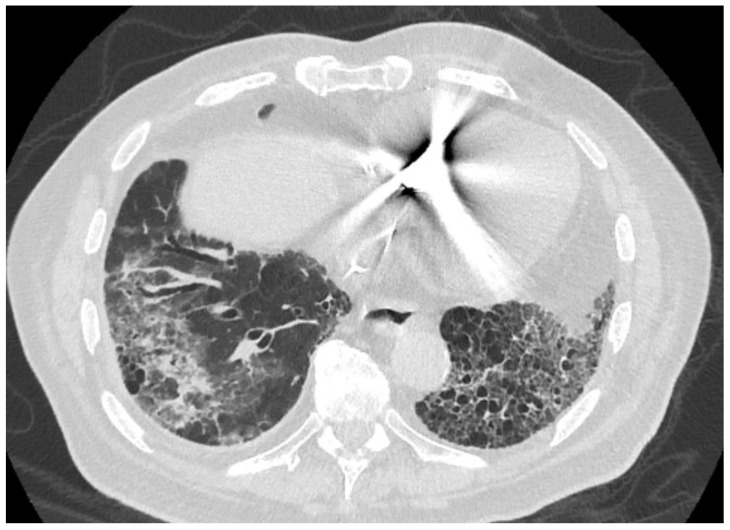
Axial view of chest computed tomography (CT) demonstrates typical honeycombing in the left lower lobe and traction bronchiectasis in the right lower lobe. The groundglass and consolidative opacity seen in the right lower lobe—which is not common in UIP/IPF—has been attributed to possible mild focal alveolar hemorrhage in the setting of thrombocytopenia or microaspiration inflammatory changes.
